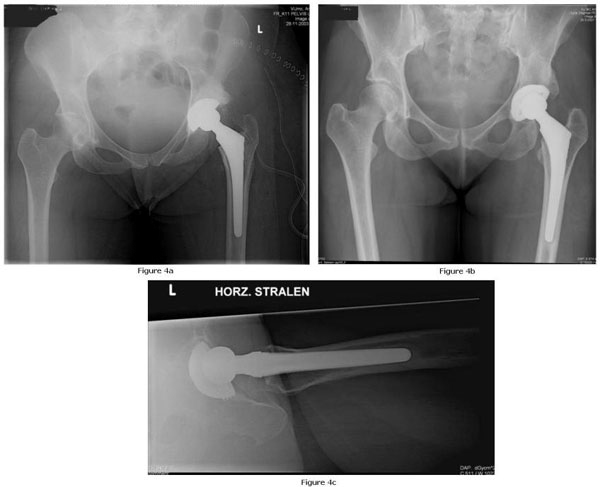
(a) Postoperative radiographs of the pelvis at 6-months follow up shows good position of hip prosthesis. (b) Postoperative radiograph of the pelvis at 40 months follow up shows further remodeling of acetabular bone stock. (c) Postoperative radiographs lateral film at 40 months follow up shows good position of the hip prothesis
